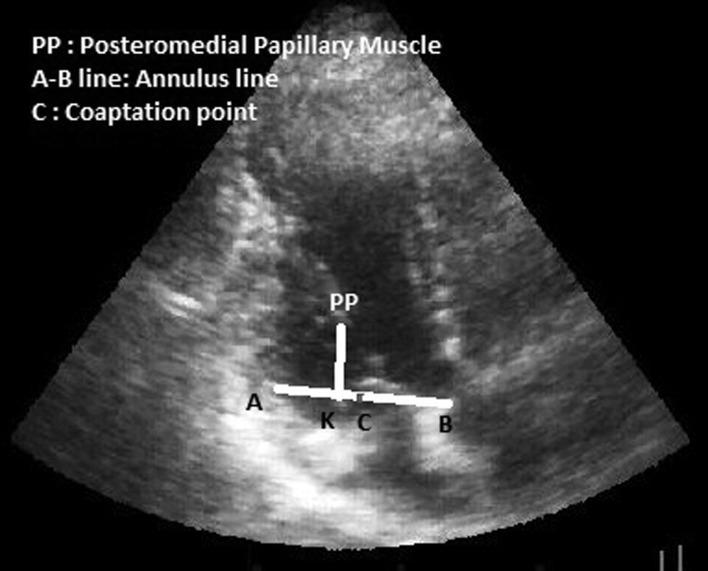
Apical Long Axis View in Transthoracic Echocardiography
